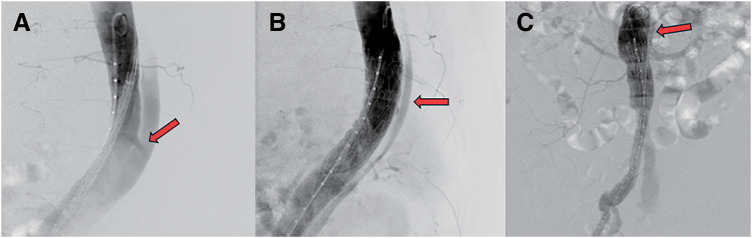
Fig. 2 Intraoperative angiography and TEVAR procedure. (A) Intraoperative angiography of the descending thoracic aorta demonstrated a large primary entry tear with contrast flow into the false lumen (arrow). A thoracic stent graft was deployed to suppress false-lumen perfusion. (B) Angiography of the same region after stent graft deployment revealed residual retrograde flow into the false lumen from the distal side (arrow). (C) Selective angiography of the abdominal visceral arteries demonstrated a re-entry tear near the left renal artery (arrow), consistent with the CT findings. No contrast flow into the AAA was observed. TEVAR: thoracic endovascular aortic repair; CT: computed tomography; AAA: abdominal aortic aneurysm
